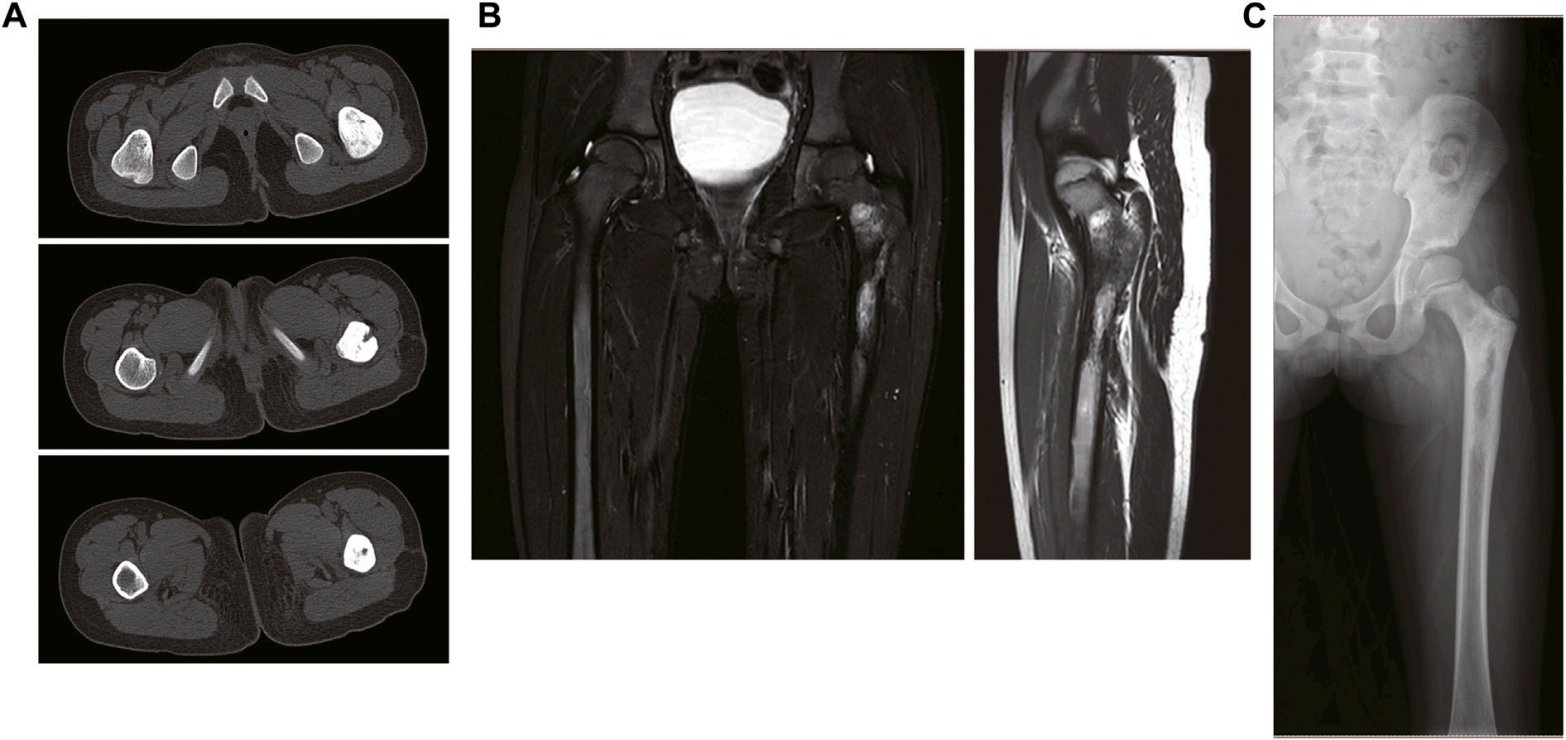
The imaging data of the patient on admission. (A) The CT results of the patient. (B) The MRI results of the patient. (C) The X-ray results of the patient.
